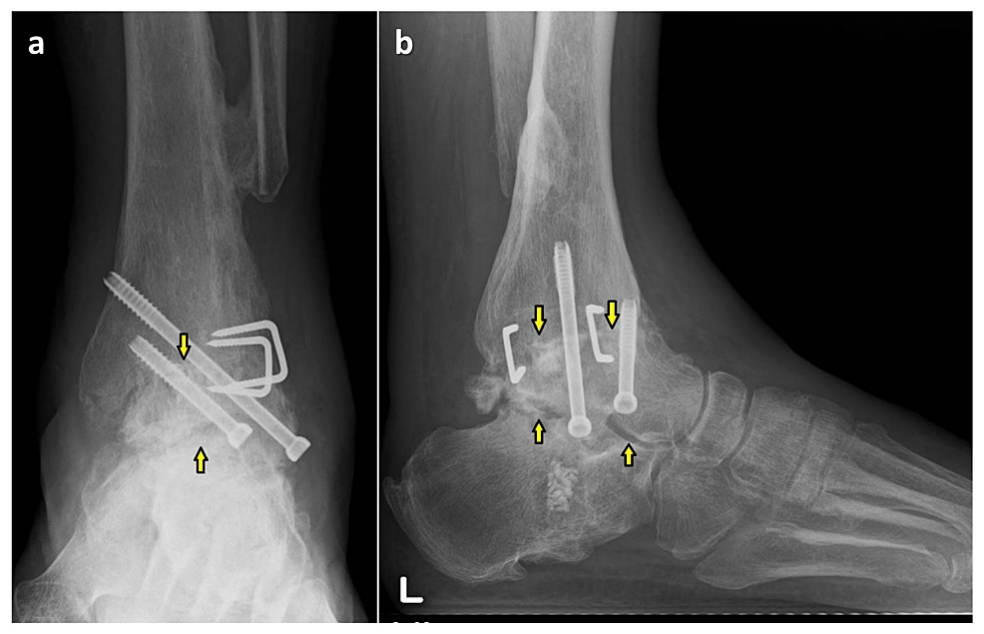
Plain radiograph at one year and three months after surgery (a) anteroposterior and (b) lateral images
